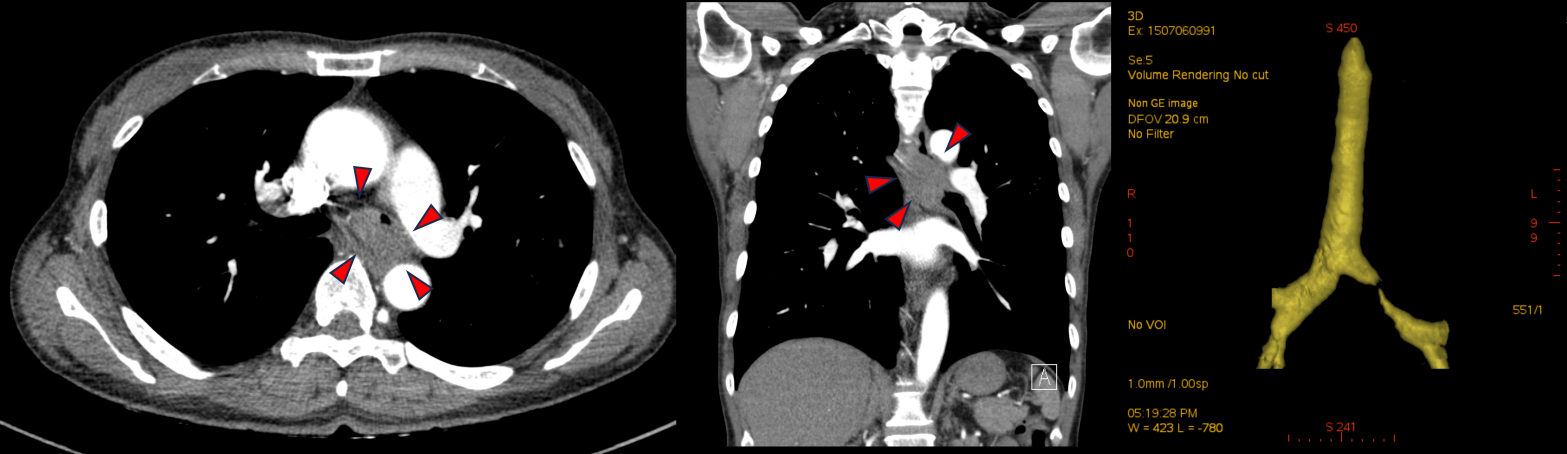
Pre-treatment chest computed tomography (CT) scan. CT scan revealed a 3.5-cm enhancing mass in the left main bronchus, causing significant luminal narrowing and impending obstruction. The mass was also abutting the thoracic aorta, raising suspicion of a possible malignancy.
